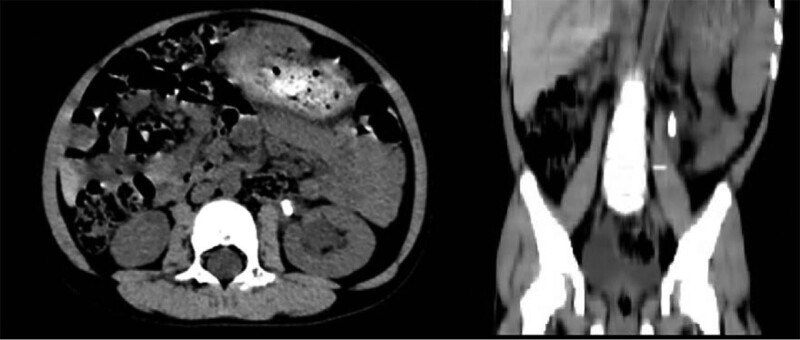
Sagittal and coronal view of lower abdominal CT before operation (Case 2). CT = computed tomography.
